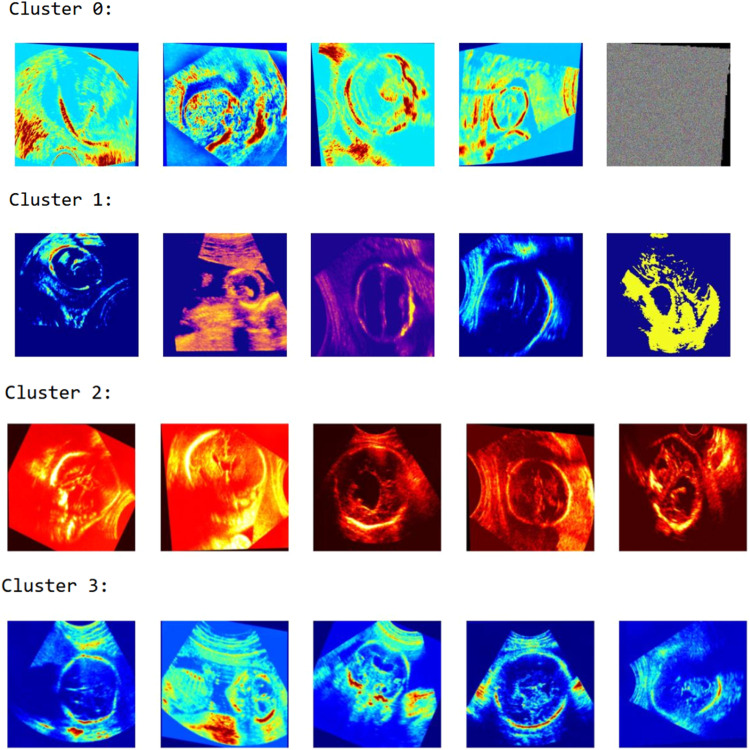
Ultrasound image clustering results using K-means (k = 4).
